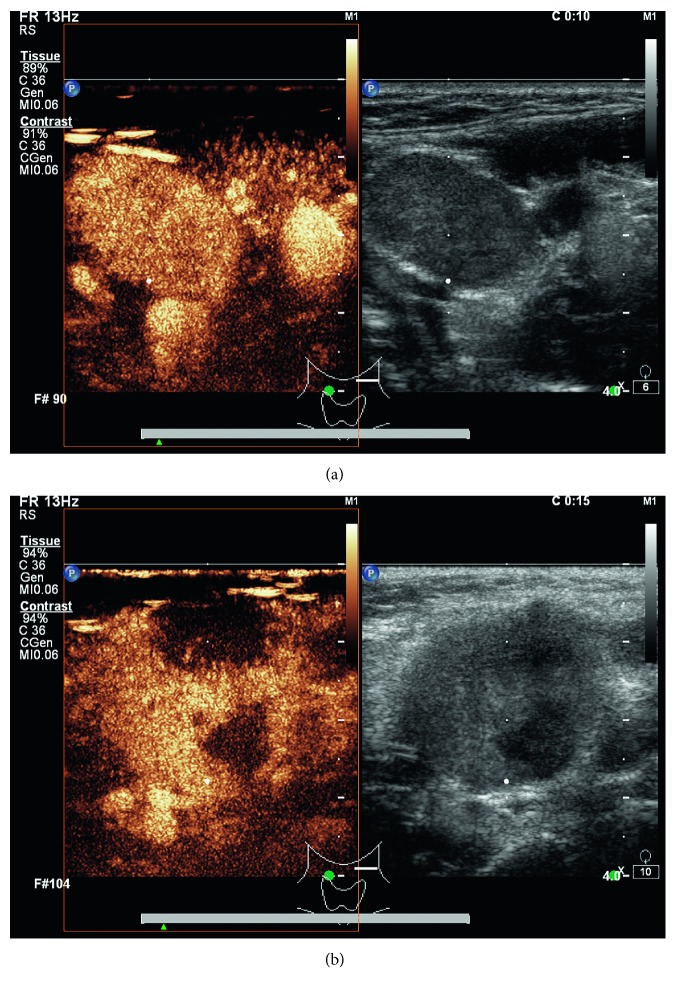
Sonogram obtained lymph nodes with a L9-3 high-frequency linear array probe (30 MHz) from different therapeutic states of lymphoma: (a) a lymphomatous lymph node with rapid heterogeneous hyperenhancement pattern; (b) a lymphomatous lymph node with rapid well-distributed hyperenhancement pattern.
